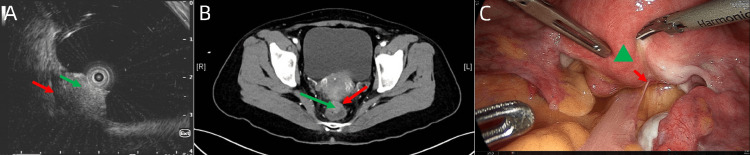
Patient’s images (endoscopic ultrasound). (A) Endoscopic ultrasound demonstrated a tumor (green arrow) invading the muscular layer of the rectum (red arrow). (B) An abdominal CT demonstrated an exophytic nodular 1.7 × 2.1 cm mass narrowing the colonic lumen (green arrow) with an intact colonic serosa (red arrow). (C) Intraoperative photo shows the posterior uterine wall (green triangle) was invaded by the tumor (red arrow).
